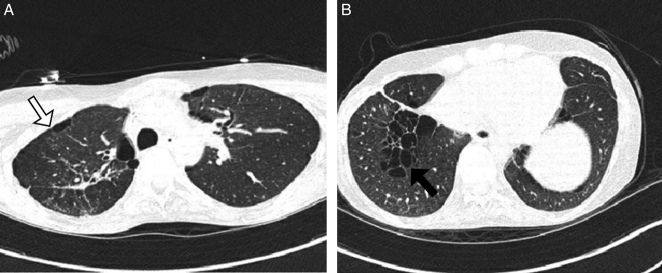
Chest computed tomography (CT) scan images for a male patient with Birt-Hogg-Dube disease: (A) White arrow indicates loss of right lung volume after apical pneumonectomy with compensatory hyperinflation of the left side; (B) Black arrow indicates diffuse irregular shape thin wall cystic changes that are more predominant in the right lower lobe, along with peri-mediastinal and peri-bronchovascular distribution.
